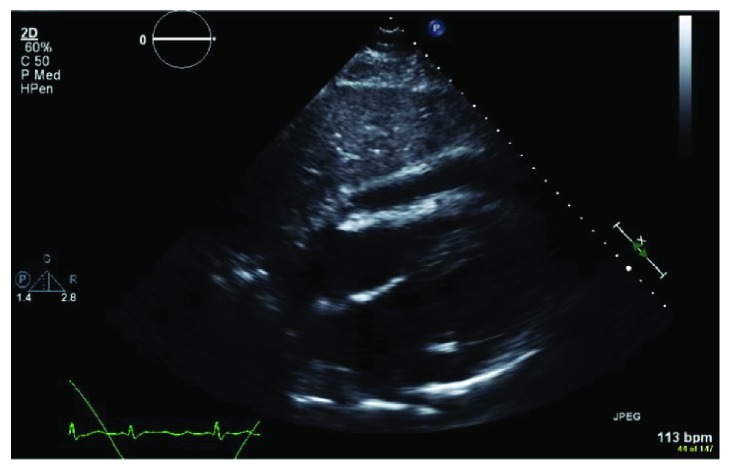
Transthoracic echocardiogram, subcostal view.
